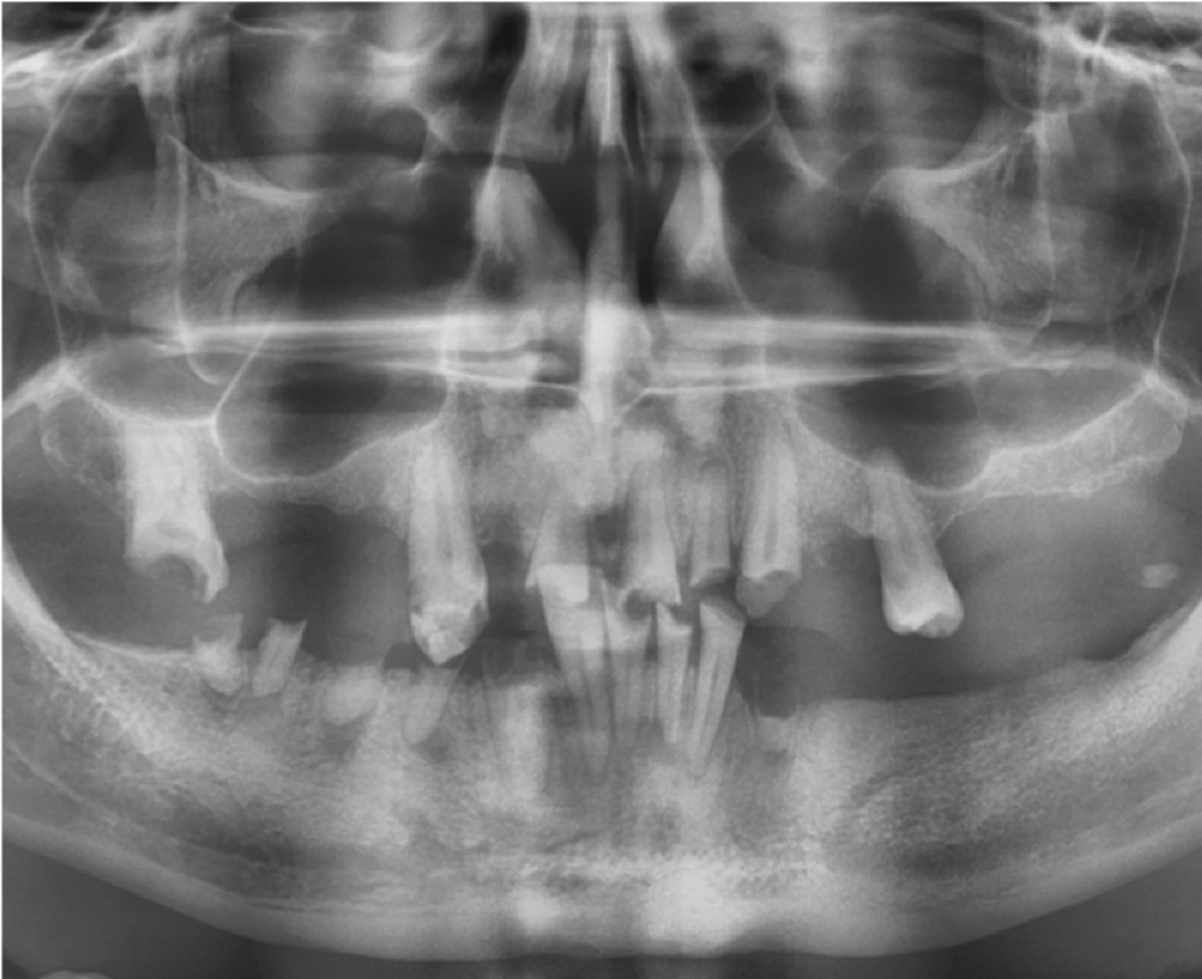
Preoperative radiograph of the treated case.
